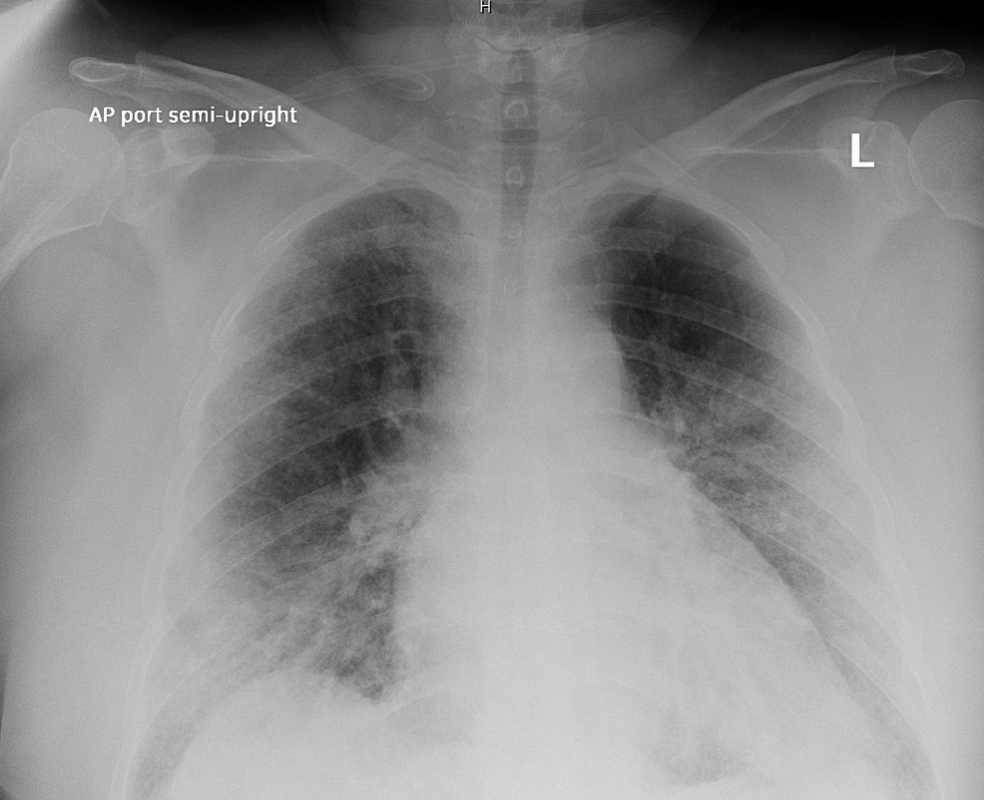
Chest radiograph with bilateral ground-glass opacities, characteristic of COVID-19 pneumonia
